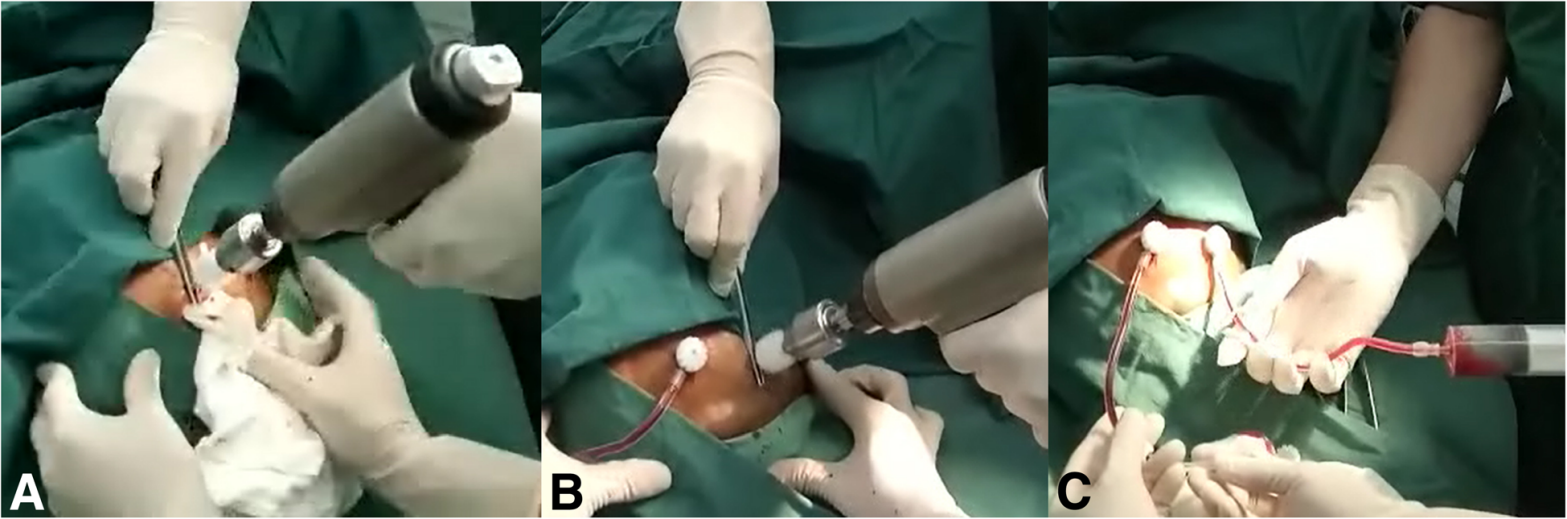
The two target points were established in the area according to the brain CT scan, which were located in the maximum level of the hematoma, and chosen to prevent injury to superficial temporal artery and its branches and middle meningeal artery (a-b). After the inner needle was retrieved, bloody fluid, which was not coagulable, could be extracted from the subdural regions respectively (c)
